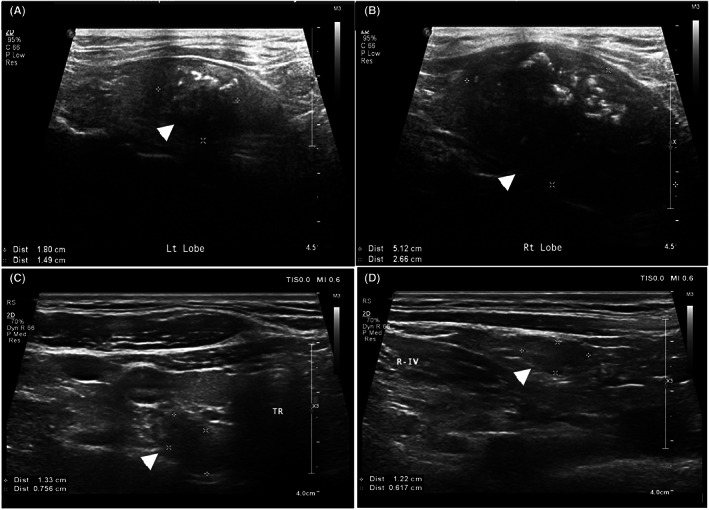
Characteristic ultrasonic images of MTC tumors and lymph nodes. (A) Longitudinal section of a hypoechogenic tumor located in the left lobe, with irregular contour, ill‐defined margin and internal calcifications (arrowhead). (B) Another hypoechogenic lesion with similar features identified in the right lobe of the same patient (arrowhead). (C) Suspicious lymph node (arrowhead) detected in the right central compartment near the trachea (TR), with unclear boundary between the nodal cortex and medullary. (D) Enlarged lymph node with internal calcification detected in region IV of the right neck (R‐IV), as indicated by the arrowhead.
